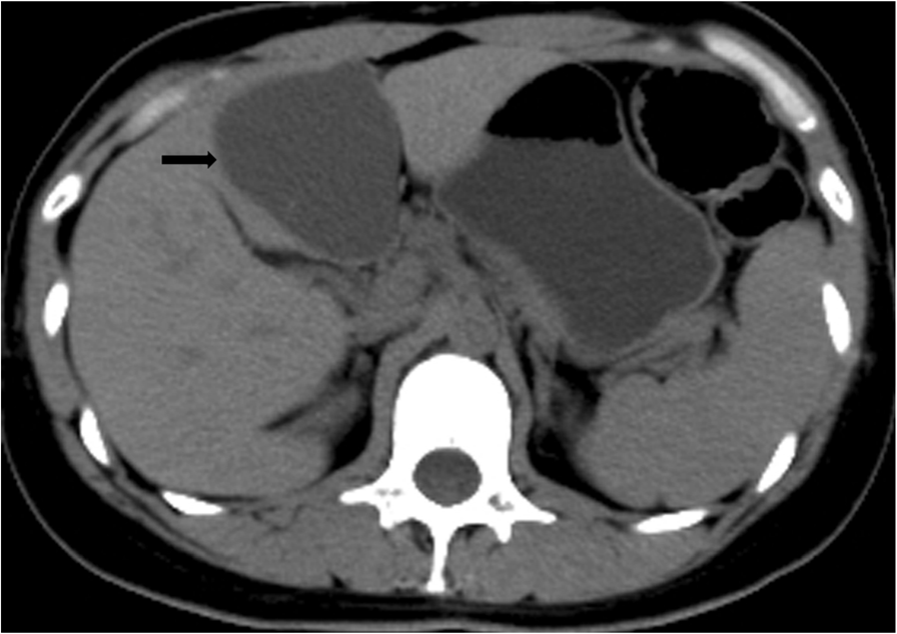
Hepatic CT scan showing a well circumscribed cystic lesion of 6.5 × 6.0 cm located in segments III of the left liver lobe.
